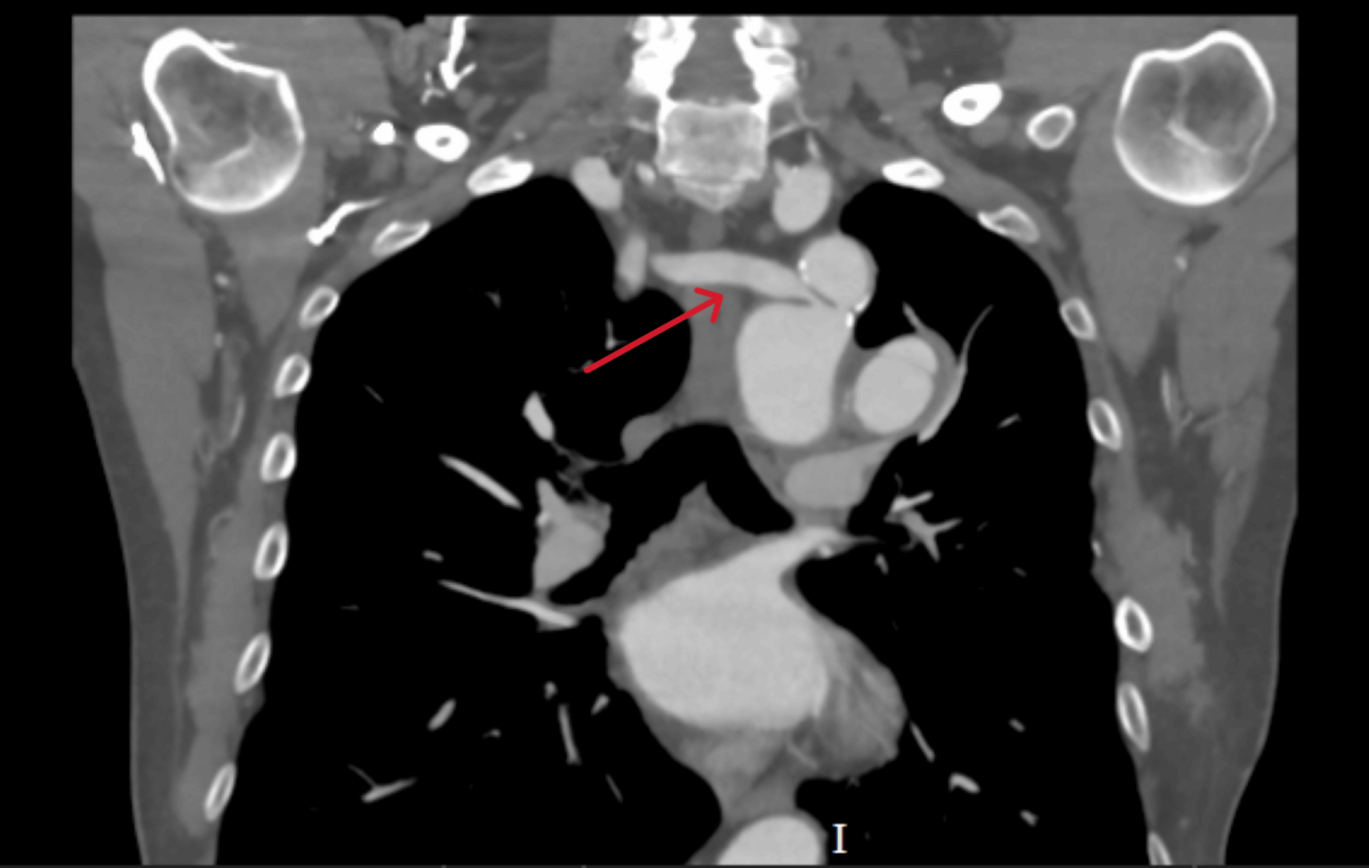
Coronal CT image showing an anomalous right subclavian artery (red arrow) arising from the aorta, distal to coarctation. CT: computed tomography
